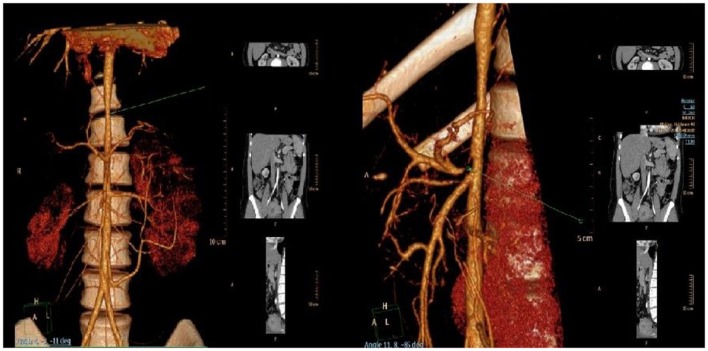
Narrowing of the upper part of the abdominal aorta (left) and critical stenosis of coeliac trunk (right) visualized by CT angiography.
